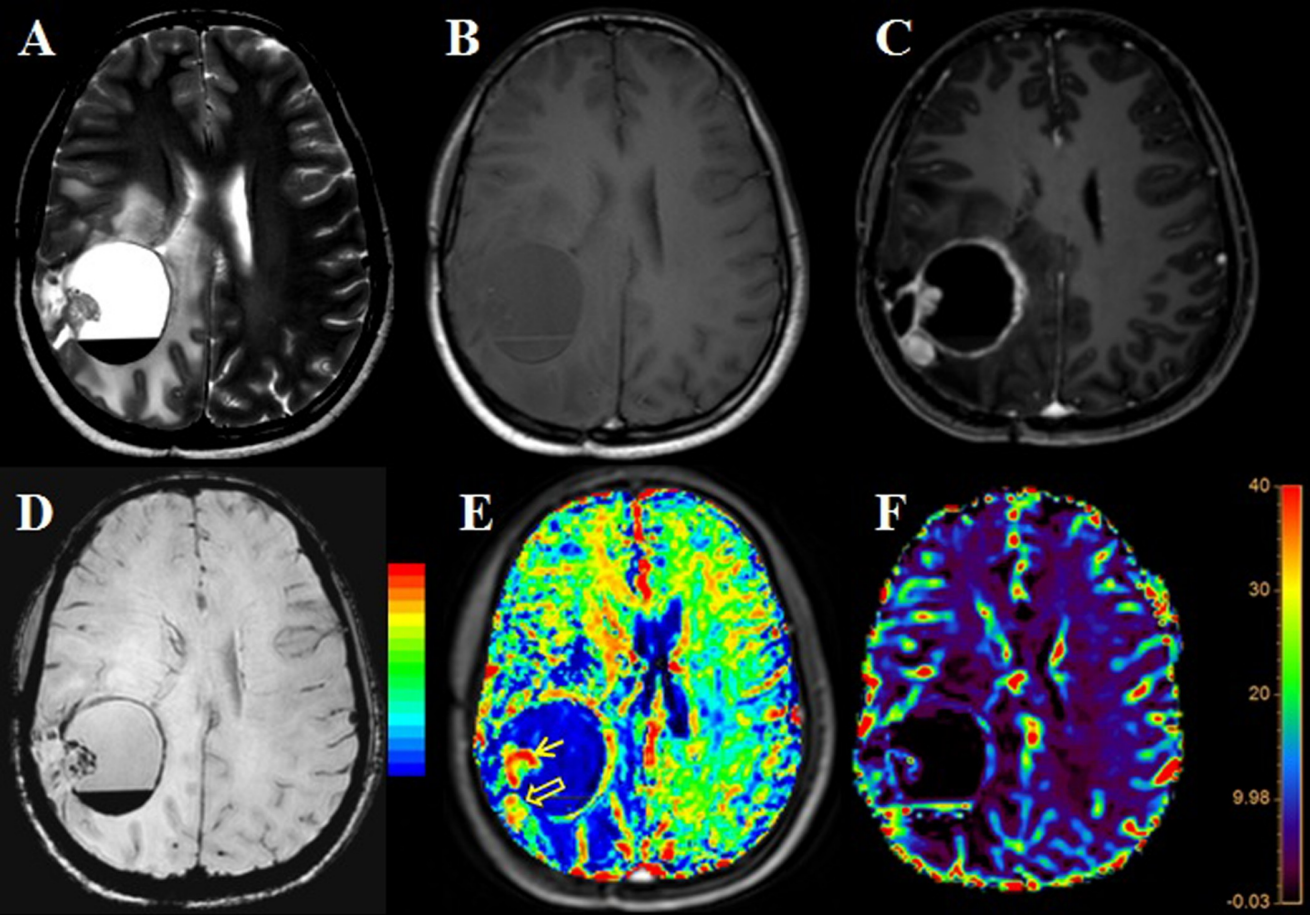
A post-operative case of high-grade glioma showing recurrent tumor with foci of susceptibility in the mural nodule as well as blood fluid level in the cystic component (E, open arrow). The mural nodule is showing high CBV on T1- perfusion (E, thick arrow). The corresponding area shows relatively low CBV on T2* perfusion MRI. Another foci with increased CBV seen posteriorly detected well on both T2*- and T1- perfusion MRI (E and F, thin arrows). Artifactual increased CBV is seen within the cystic component of the tumor on T2*- perfusion (F, thin arrow).
